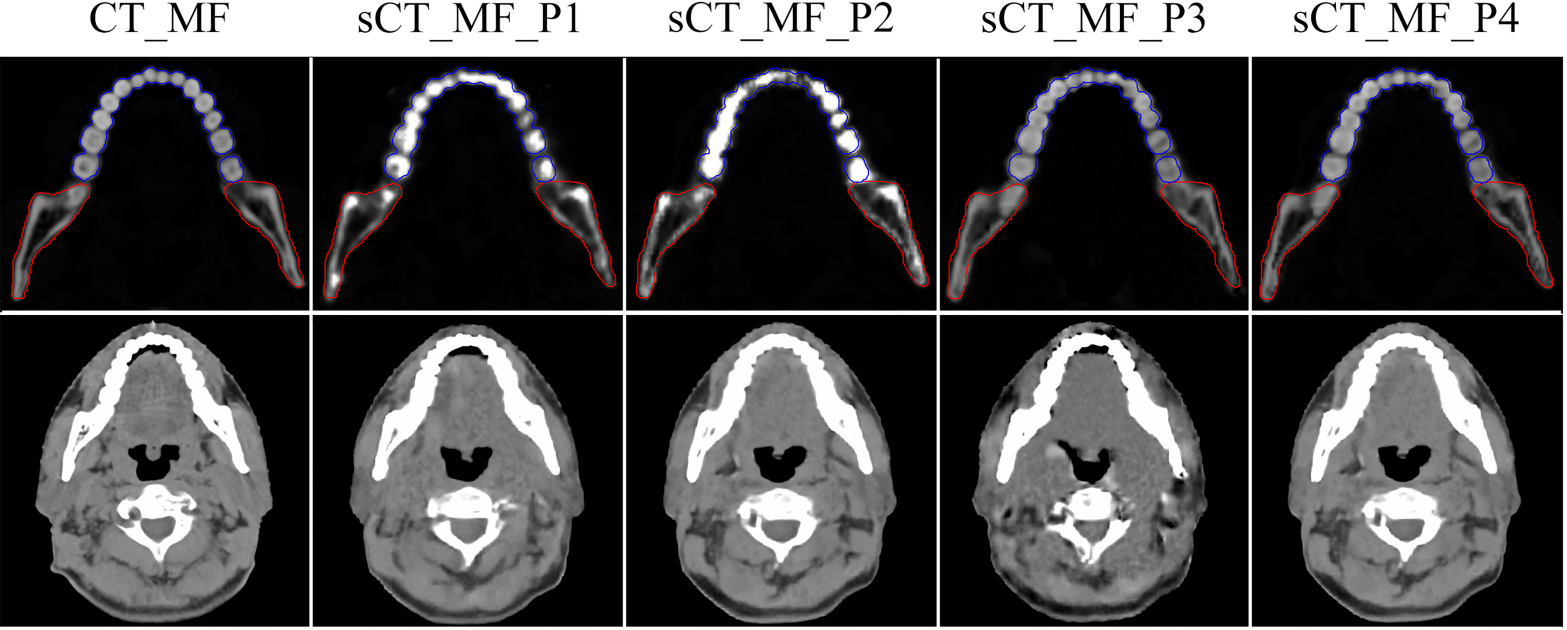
Visualized differences of CT_MF and sCT_MF images with different preprocessing methods. The display windows for the first and second rows were [0, 3000] HU and [-360, 440] HU, respectively. Blue lines represent the contour of the teeth, and red lines represent the contour of the mandible. The images in the first to fifth columns were CT_MF, sCT_MF_P1, sCT_MF_P2, sCT_MF_P3 and sCT_MF_P4, respectively. Different image preprocessing methods have their own advantages in different CT number ranges, and sCT_MF_P4 performs best.
